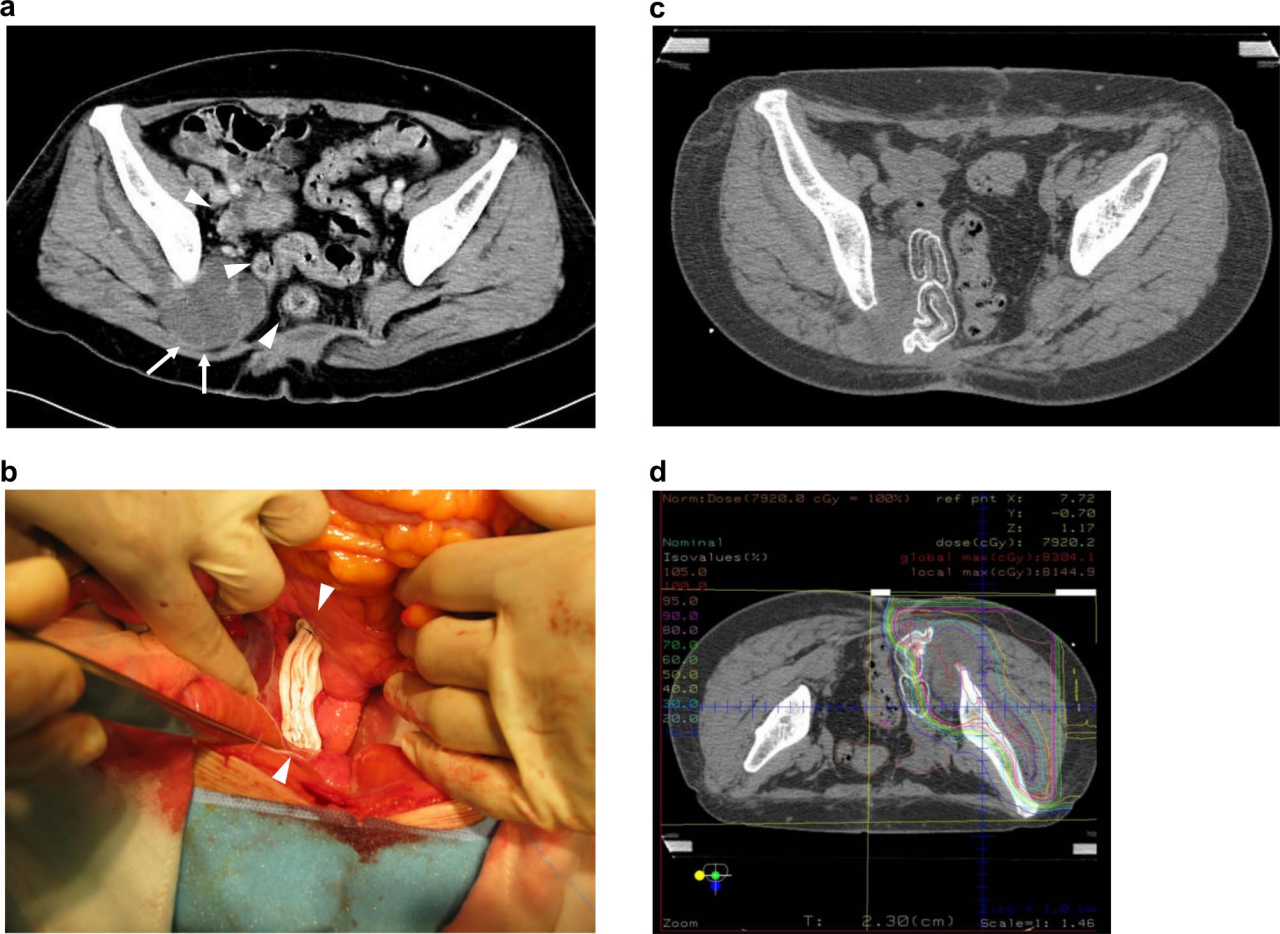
Images of a representative patient who underwent expanded polytetrafluoroethylene (ePTFE) spacer placement surgery. a Recurrence of chondrosarcoma (white arrow) broadly abuts the small intestine, sigmoid colon, and rectum (arrowhead). b Operative findings show that the ePTFE spacer (arrowhead) was inserted and sutured to the surrounding tissue at the left side of the tumor. c Postoperative computed tomography reveals that sufficient space between the tumor and the gastrointestinal tract was maintained by the ePTFE spacer. d The treatment plan shows that the clinical target volume was totally irradiated at more than 90% of the prescribed dose, and gastrointestinal tract was barely irradiated
